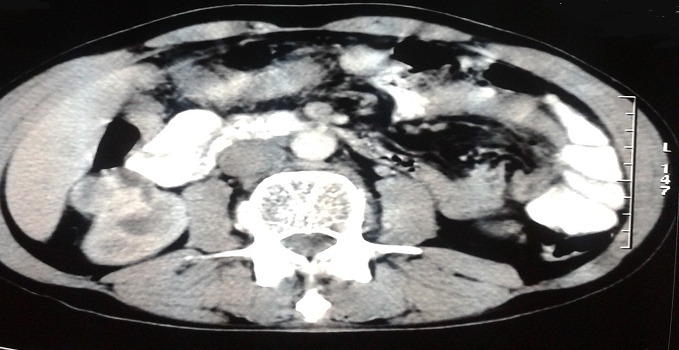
CT scan of the abdomen showing a solid tumor mass in the right kidney measuring 34mm with exophytic development and moderate enhancement
